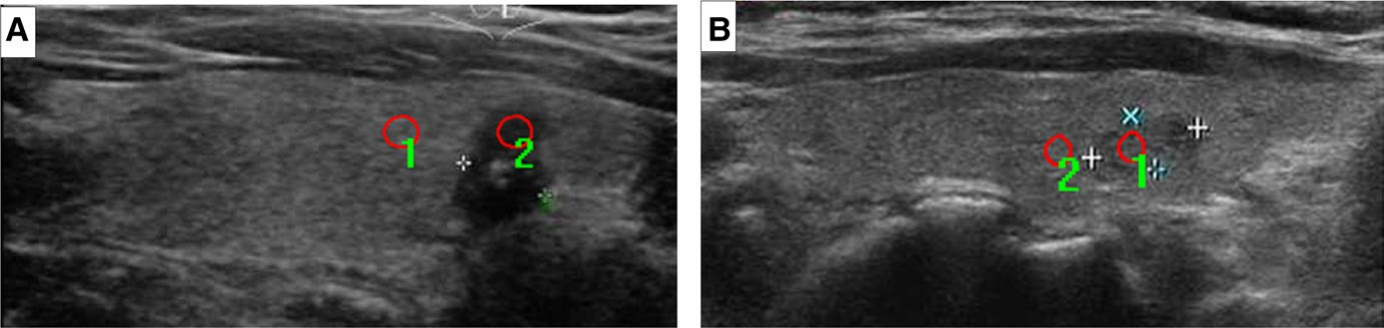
For PTCs with heterogeneous echogenicity (A), regions of interest (ROIs) are drawn to include the nodule (1) and the surrounding normal thyroid tissue (2). The mean gray scale values for the PTCs and the surrounding normal thyroid tissue were 31.9 and 83.8, respectively, and the UGSR was 0.381 (31.9/83.8). For NGs with heterogeneous echogenicity (B), regions of interest (ROIs) are drawn to include the nodule (1) and the surrounding normal thyroid tissue (2). The mean gray scale values for the NGs and the surrounding normal thyroid tissue were 74.7 and 84.9, respectively, and the UGSR was 0.879 (74.7/84.9). Note that the ROI (red cycle) should be drawn around the largest area of the echo‐dominated region of heterogeneous echogenicity nodules. PTCs, papillary thyroid carcinomas; NGs, nodular goiters; UGSR, ultrasound gray scale ratio
